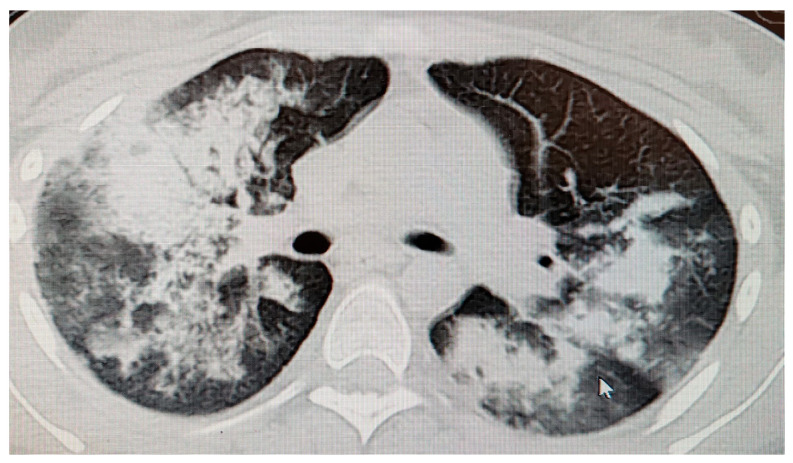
Chest computed tomography (CT) demonstrates bilateral ground-glass opacities with a perihilar predominance and areas of consolidation in the lower lobes, consistent with diffuse alveolar hemorrhage in the context of AAV-associated pulmonary hemorrhage. Case 1 (PR3-ANCA-positive GPA), 15-year-old female, after cycle VI of cyclophosphamide therapy.
